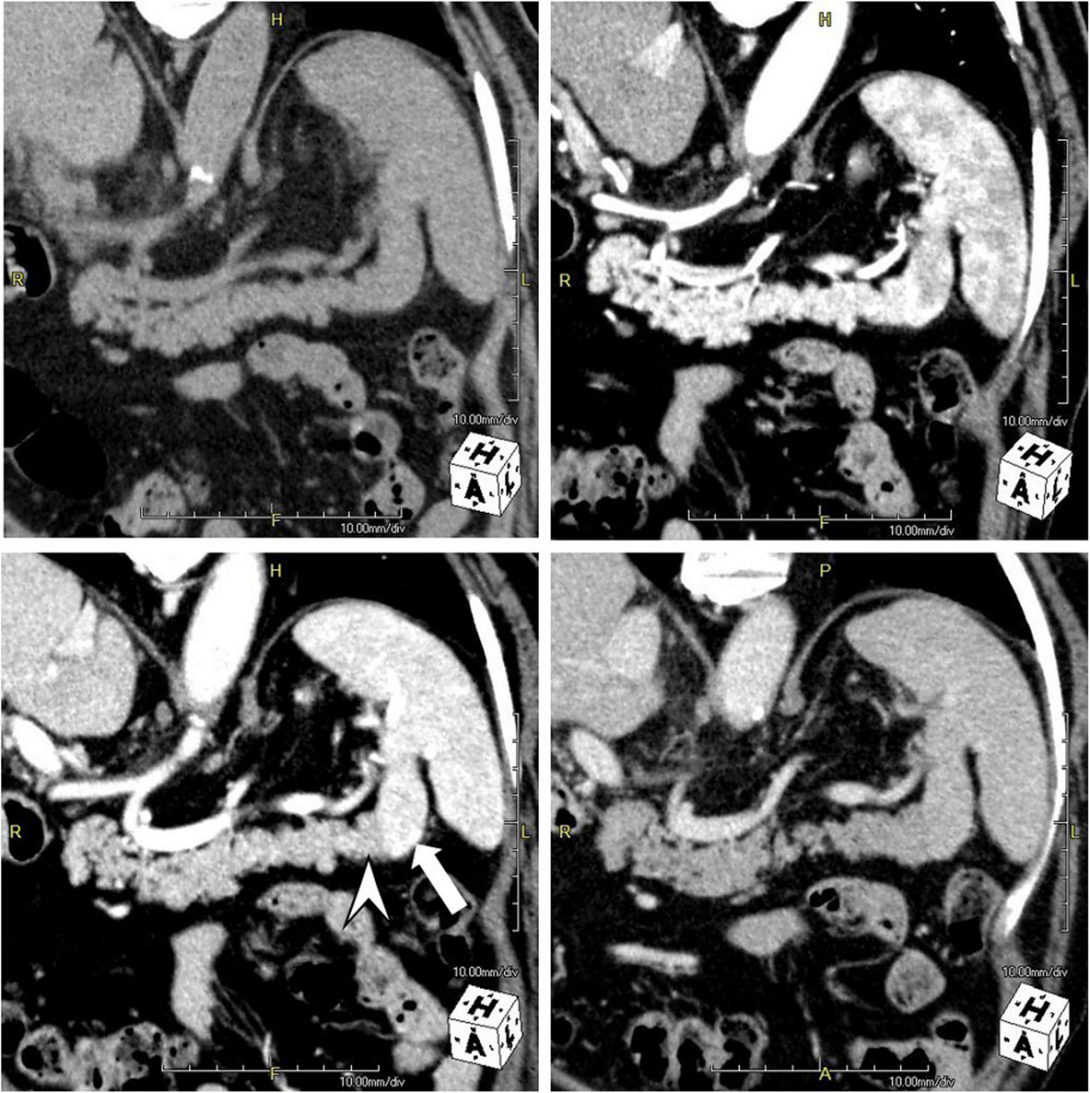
Coronal multiplanar reconstructed images of triple-phase contrast-enhanced multidetector-row computed tomography show fusion of the pancreatic tail (*arrowhead*) to the lower medial pole of the spleen (*arrow*), resulting in its bi-loaded configuration
